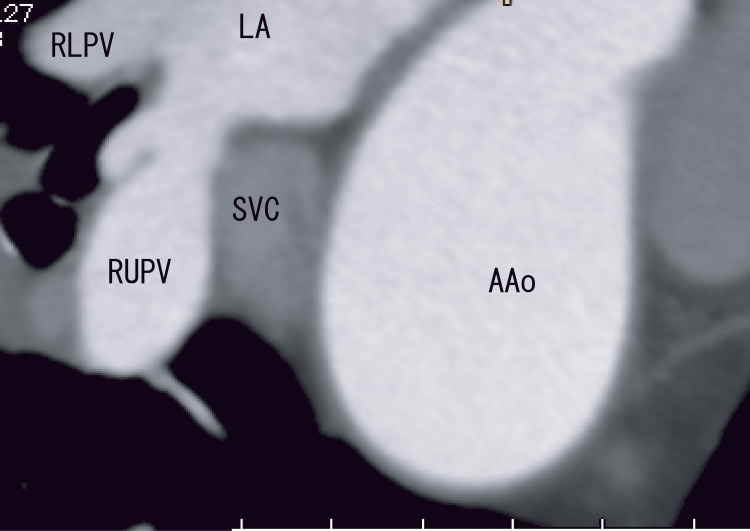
Oblique CT images showing the AAo and SVC Oblique enhanced CT images clearly revealed no cleft, no linear white thrombi, and no RUPV thrombi. There were a few changes in the walls between the SVC and AAo. The SVC has a vague boundary. The angle of these images is similar to that of the TEE images. AAo: Ascending aorta; LA: Left atrium; RLPV: Right lower pulmonary vein; RUPV: Right upper pulmonary vein; SVC: Superior vena cava.
